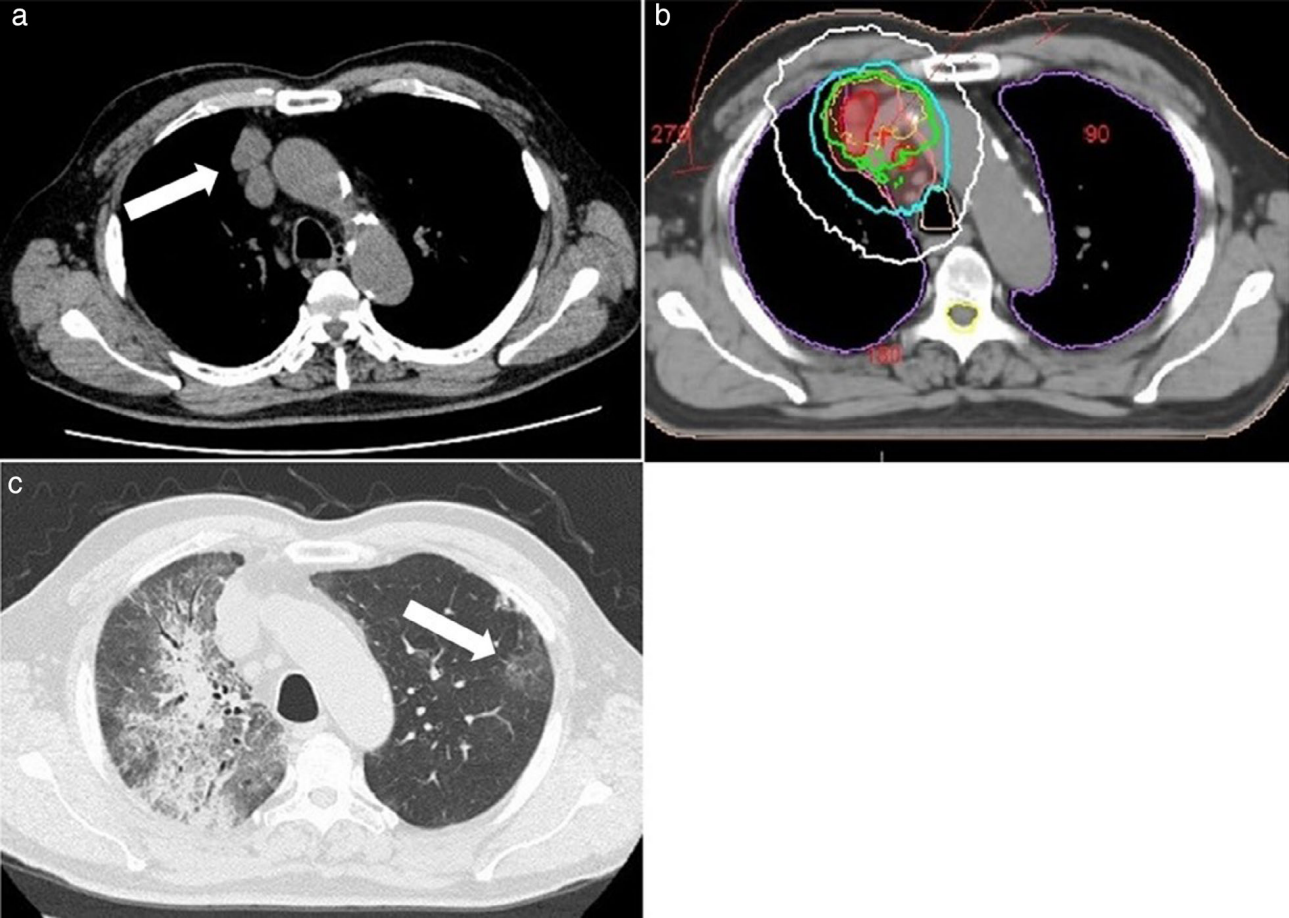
(a) Computed tomography (CT) shows the recurrence of mediastinum lymph node metastases (arrow) in a man in his 70s. (b) He received three‐dimensional radiotherapy; isodose lines of 100% (yellow), 95% (light green), 85% (light blue), 50% (white), and the planned target volume (pink) are shown. (c) After one course of an anti‐PD‐1 antibody (2 months after thoracic radiation therapy), CT showed newly developed patchy opacity with air bronchograms in the irradiated area, which had also spread to the low‐dose area and outside the irradiated area. Ground‐glass opacity of the left lung (arrow) was also observed and diagnosed as organizing pneumonitis.
